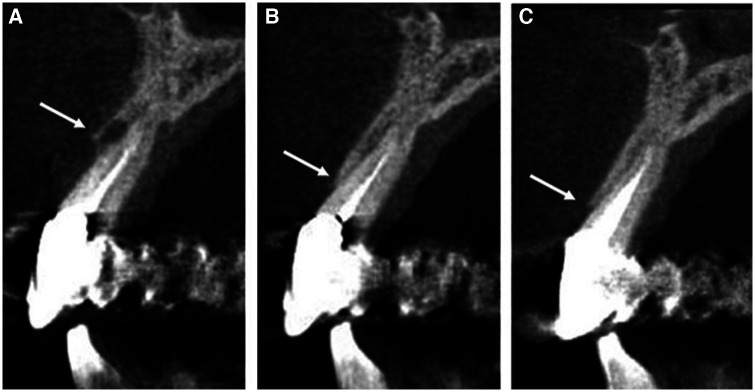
Computed tomography images showing bone levels (indicated by white arrows) at (A) initial presentation of pocketing during supportive periodontal therapy appointment with notable bone loss on the facial surface of #8, (B) 6-month follow up with visible bone regeneration and resorption of the carbonate apatite granules, (C) additional growth of regenerated bone at 18-month follow-up appointment.
